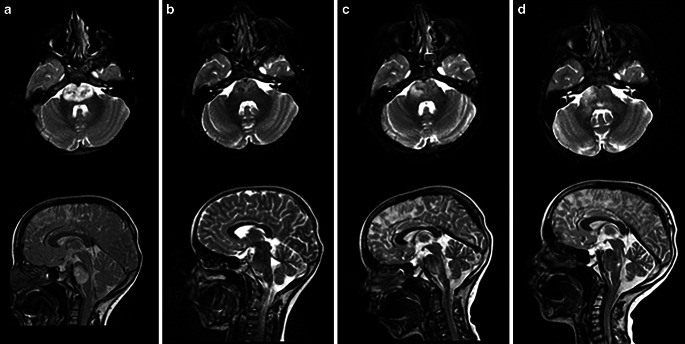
Imaging of the tumor in T2w-MRI axial and sagittal at the following timepoints: a initial diagnosis 01/2020, b 6 months after the initial irradiation, c progression 01/2021, d 1 month after the second re-irradiation
